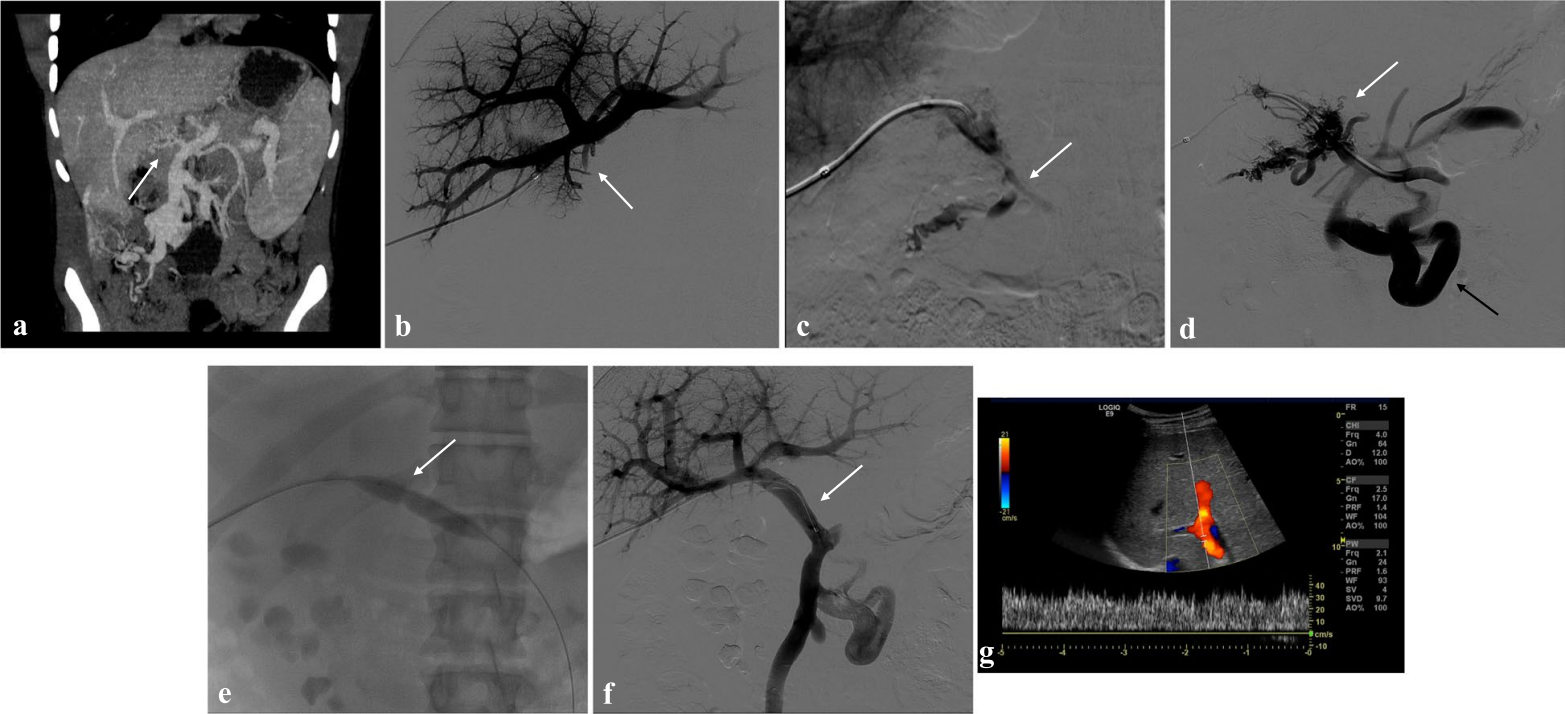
Portal vein CTO in a 16-year-old boy with a history of portal hypertension 15 years after liver transplantation (whole graft) for Alagille syndrome. a Portal phase coronal CT image shows the complete portal vein thrombosis involving the portal extrahepatic trunk with cavernous transformation (arrow). b Anteroposterior view of a percutaneous transhepatic portography image shows a regular representation of the intrahepatic portal branches. Complete occlusion of the extrahepatic portal vein at the hepatic hilum (arrow) is demonstrated. c Portography image shows the opacification of a very tiny vascular structure (arrow) which represents the main portal vein fibrotic vestige. d Inferior mesenteric portography image obtained through a 5Fr catheter which was advanced across the obstructed tract confirms the total occlusion of the main portal vein, with opacification of enlarged porto-mesenteric varices (black arrow) and portal cavernoma (white arrow). e Fluoroscopic image shows angioplasty of the main portal vein, performed through a 12-mm non-compliant balloon catheter. The focal notch (arrow) represents the tight anastomotic stenosis that probably led to secondary thrombosis. f Control portography shows a re-expanded extrahepatic portal vein (arrow) with normal opacification of intrahepatic portal branches. g Color-Doppler Ultrasound follow-up image shows regular hepatopetal flow of the main portal vein
